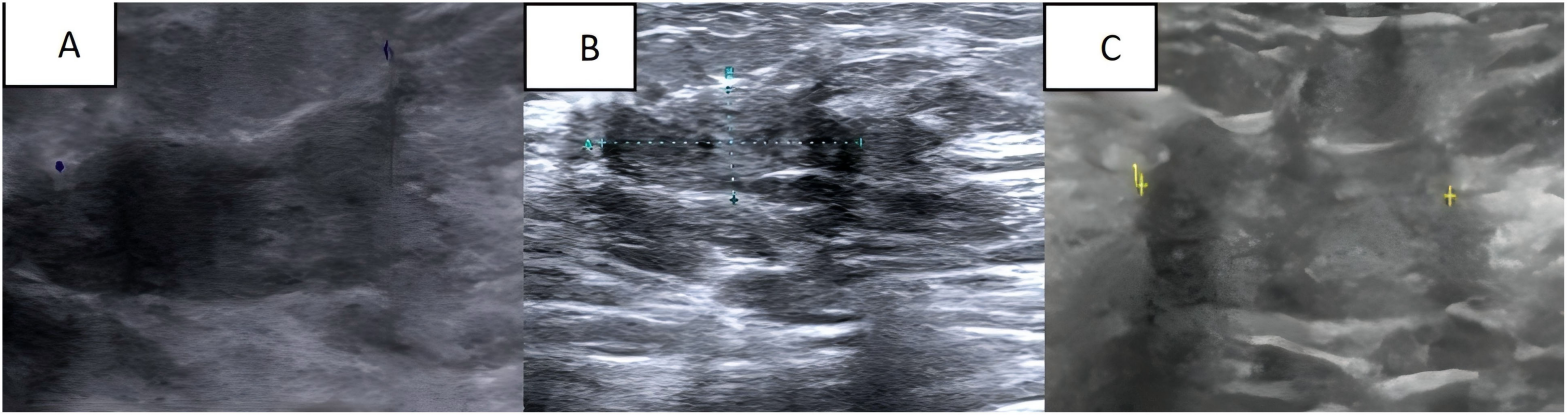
(A) Breast colour Doppler ultrasound after 4 cycles of chemical and targeted therapy; (B) Breast colour Doppler ultrasound after 8 cycles of chemical and targeted therapy; (C) The most recent follow-up breast colour Doppler ultrasound.
